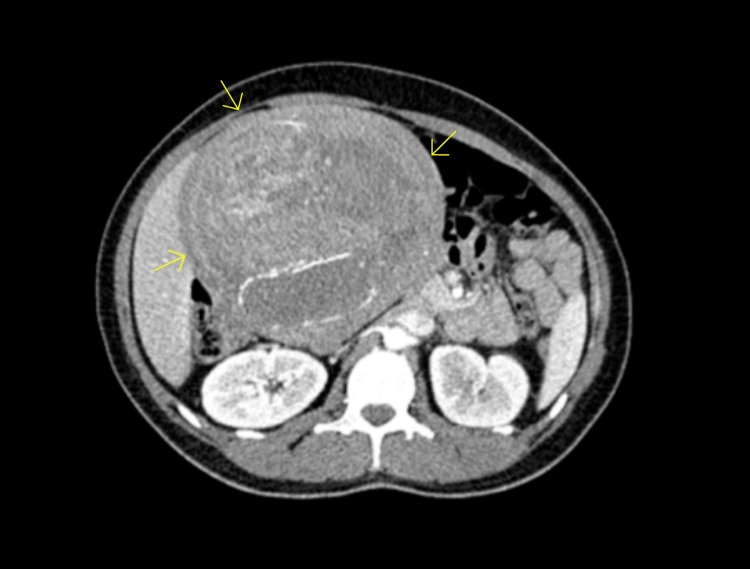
Computed tomography scan displaying an axial view of the abdomen and pelvis exhibiting an intra-abdominal/pelvic mass (depicted by the yellow arrows) appearing to originate from the uterine fundus
